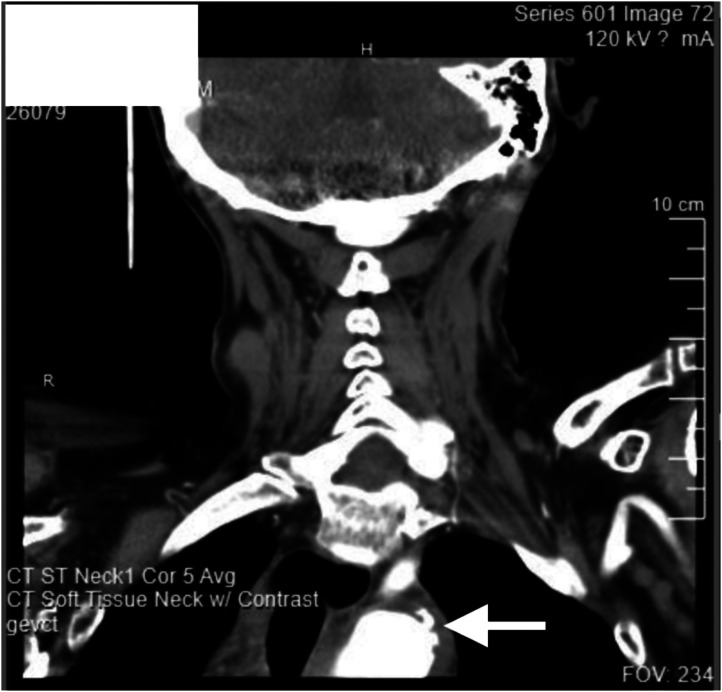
Computed tomography soft tissue neck with arrow indicating the contrast extending into the posterior wall of the proximal descending aorta consistent with a penetrating atherosclerotic ulcer in coronal view.
